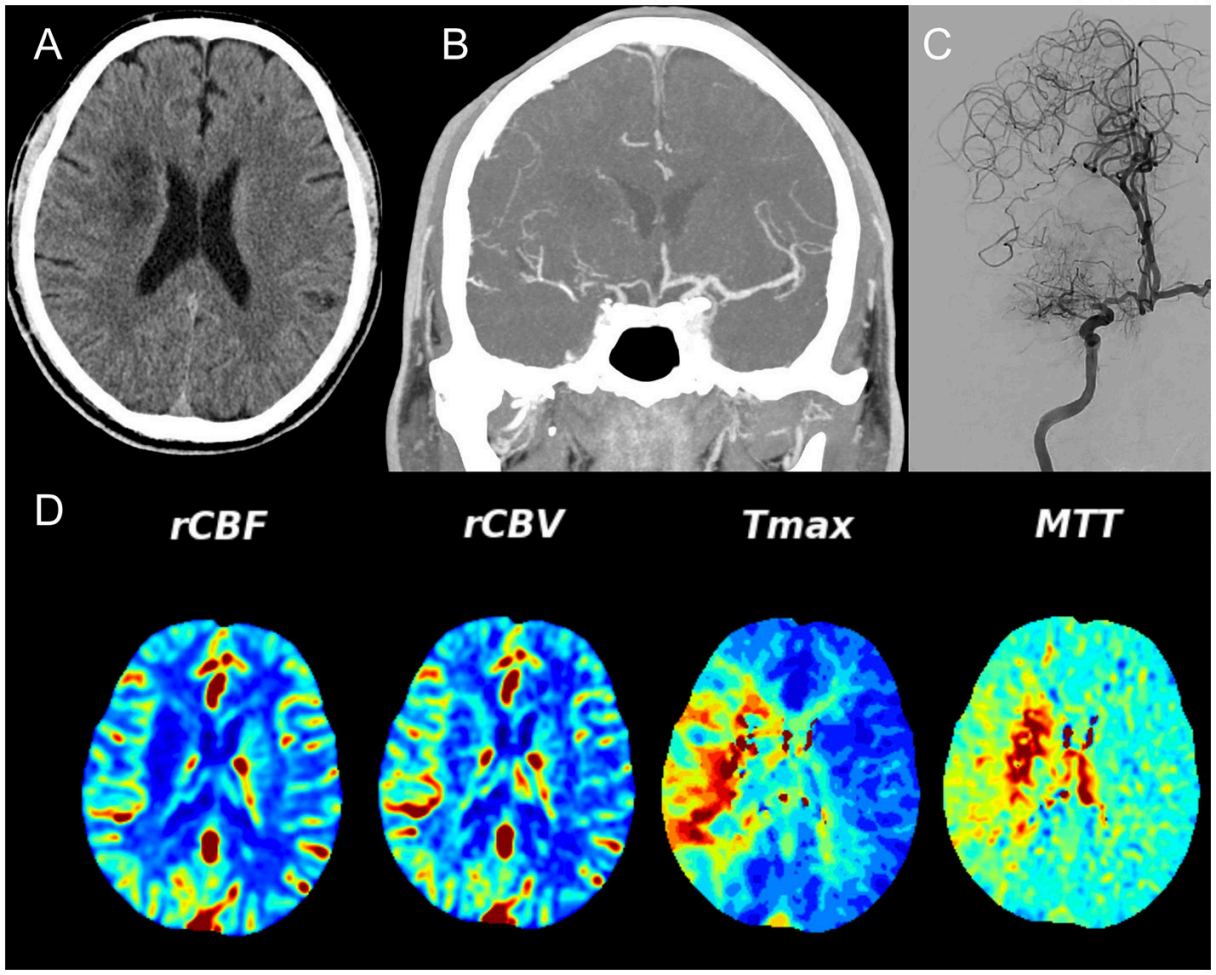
Unenhanced CT (A) demonstrates recent ischemia in a deep watershed pattern, CTA (B) in a coronal view shows a high degree MCA stenosis that is confirmed on conventional angiography (C). Note the excellent collateral network from the leptomeningeal collaterals from the ACA territory toward the MCA territory. (D) Demonstrates the CT Perfusion parameters – relative cerebral blood flow (rCBF) and — volume (rCBV) that are both still normal whereas the Time to maximum contrast (Tmax) and the mean transit time (MTT) are significantly delayed over the right hemisphere indicating hypoperfusion.
